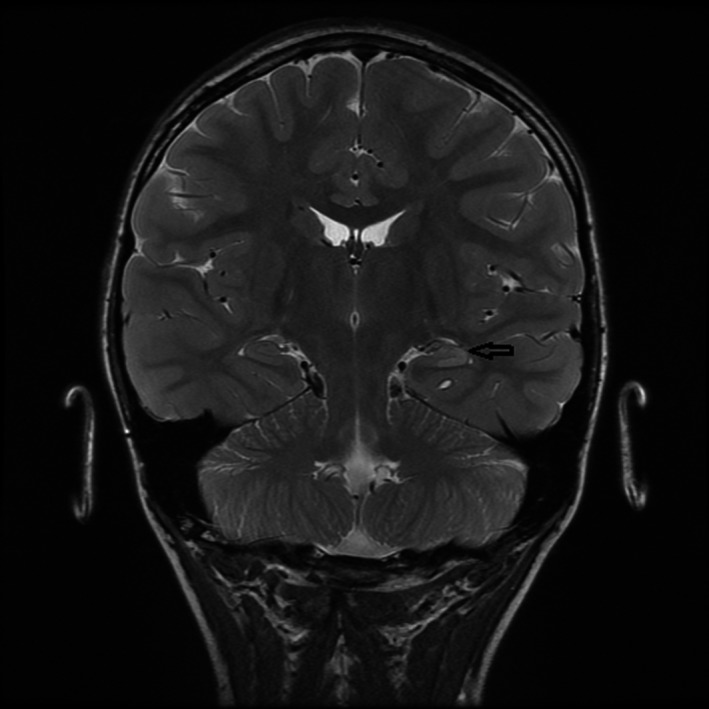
Epilepsy protocol MRI brain through hippocampi at baseline: Coronal T2 image reveal hyperintense signal in the left hippocampus (arrow). Right hippocampus is normal in signal and morphology
